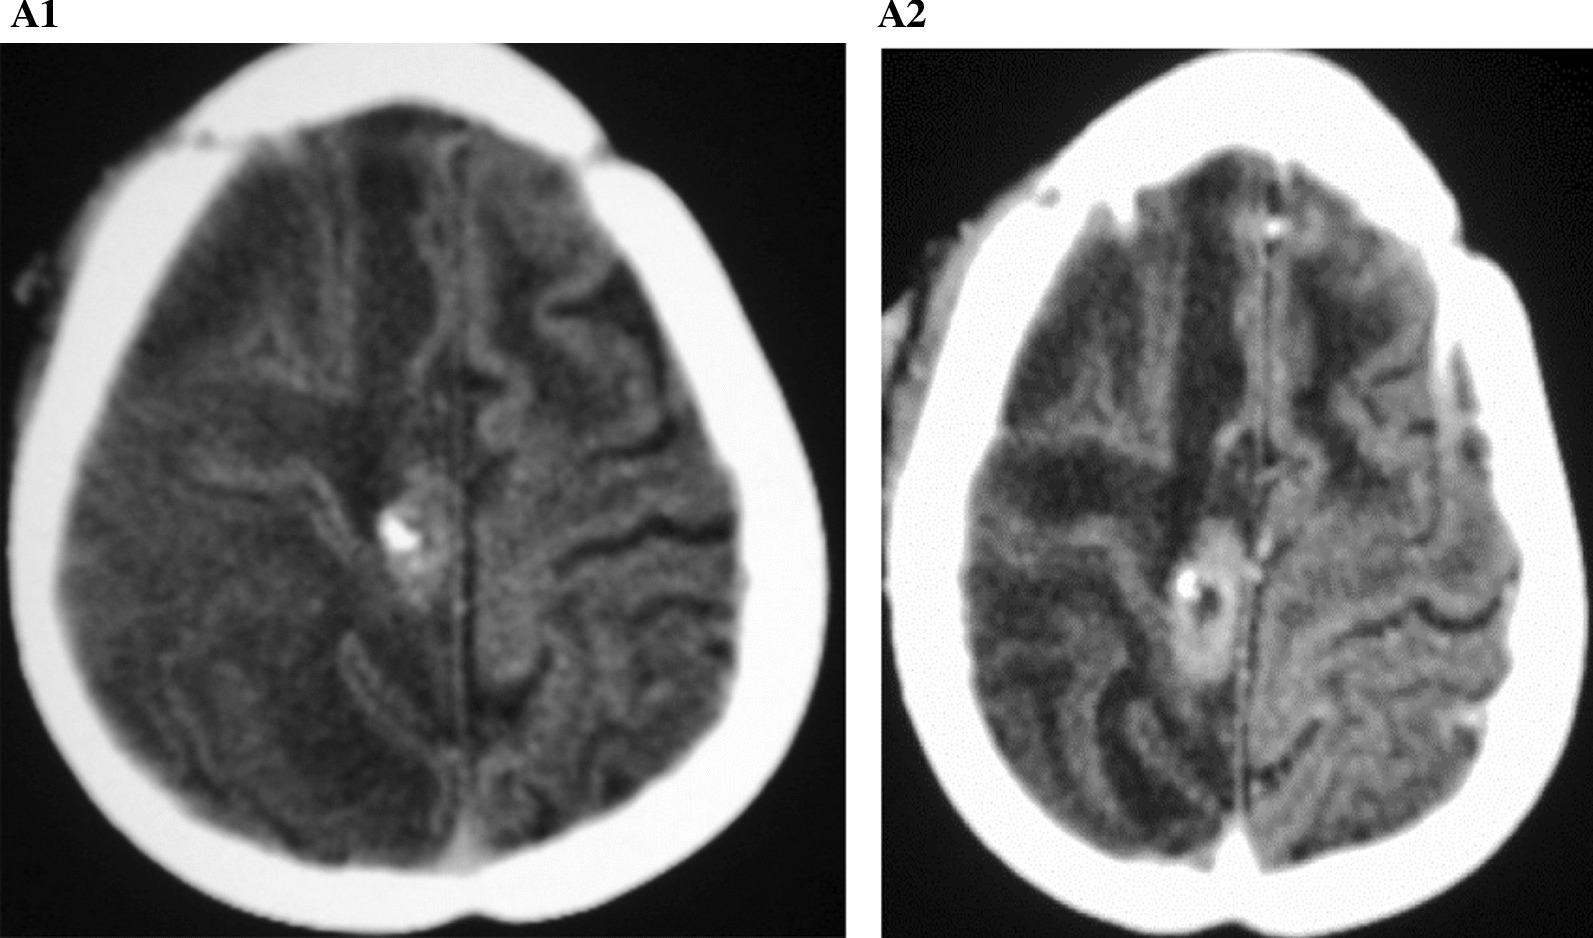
Computerized tomography scan findings of the case. A1 None contrasted axial brain CT scan shows generalized hypodensity in the right hemisphere with no white–gray matter differentiation. A calcific lesion noted in the right perifissural region. A2 Contrasted axial brain CT scan with no significant gyral enhancement
